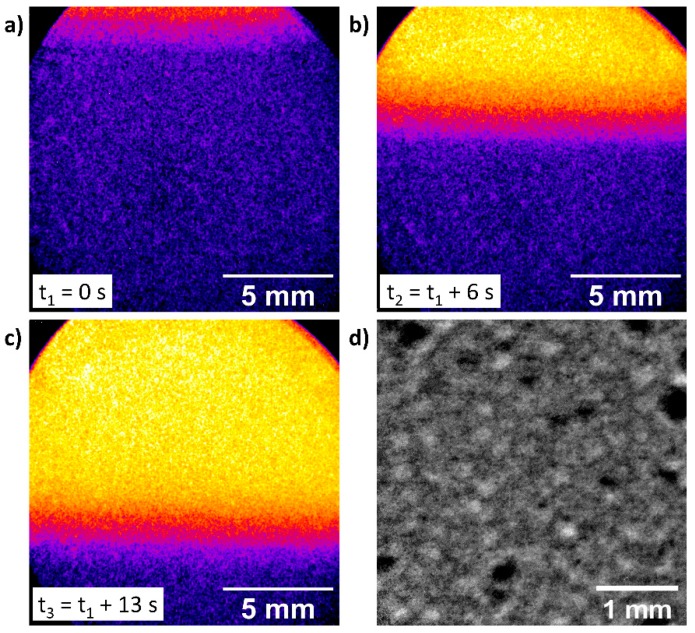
(a–c) Neutron radiography of pumping process in 2^nd^ Gen. cell; (d) in-operando neutron radiography image of ODC.
